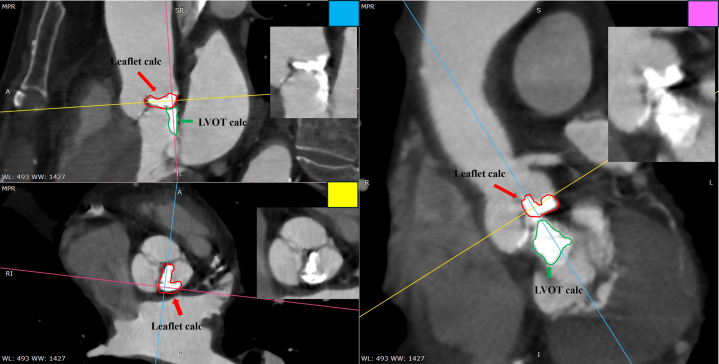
Preprocedural Computed Tomography Showing Annular-to-LVOT Calcification Preprocedural multiplanar reconstructed computed tomography images demonstrate severe calcification extending from the annulus to the LVOT. The pink, yellow, and blue lines indicate the reconstructed planes. Red arrows mark leaflet calcification, and green arrows indicate extensive LVOT calcification. LVOT = left ventricular outflow tract.
